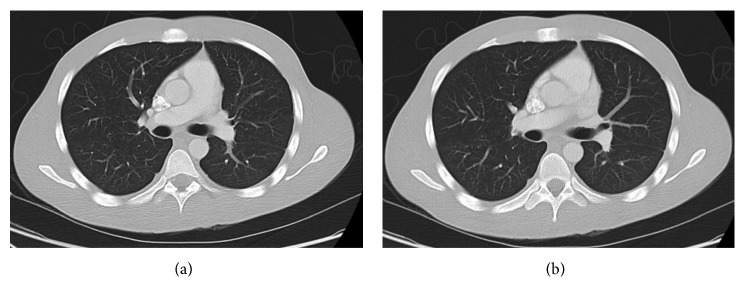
(a, b) Complete resolution of both parenchymal and pleural opacities on follow-up CT thorax following treatment.
